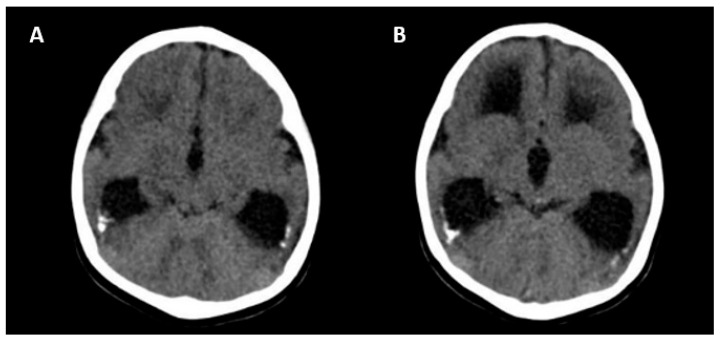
Computed tomography axial view of a newborn skull with intrauterine cytomegalovirus infection showing periventricular calcifications (A) and moderate ventriculomegaly (B).
